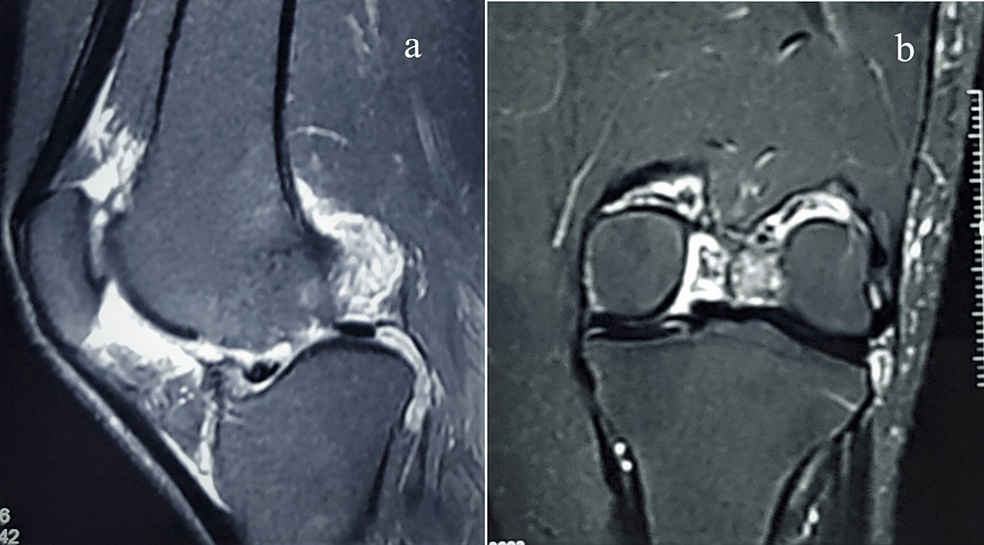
MRI of left knee in sagittal (a) and coronal (b) sections shows calcifications with deposits in synovium.
